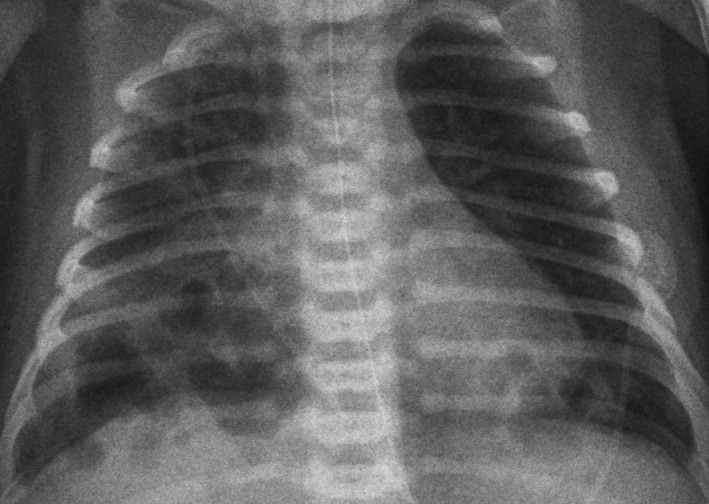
Thorax radiography. Bilateral basal condensation. Bilateral cystic thick‐walled cystic images within the condensation
